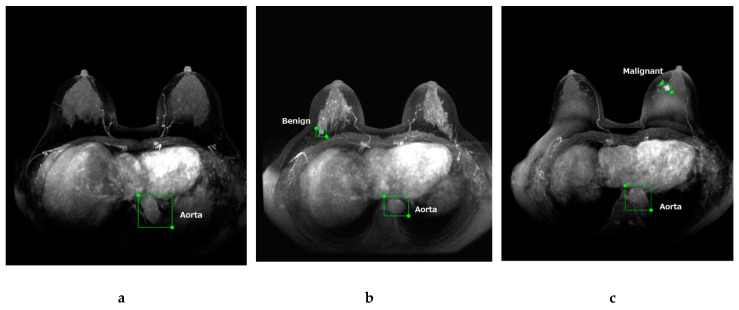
Maximum intensity projection of dynamic contrast-enhanced breast magnetic resonance images. Representative images of labeled normal (a), benign (b), and malignant (c) breast lesions.
